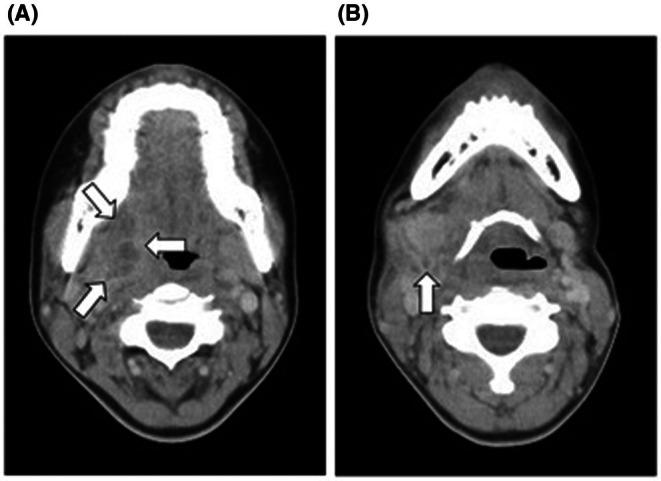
Computed tomography on the third day. (A) Low‐density area with peripheral contrast enhancement was observed. (B) A contrast defect was observed in the branch of the right internal jugular vein leading to the abscess, suggesting the presence of an intravascular thrombus.
